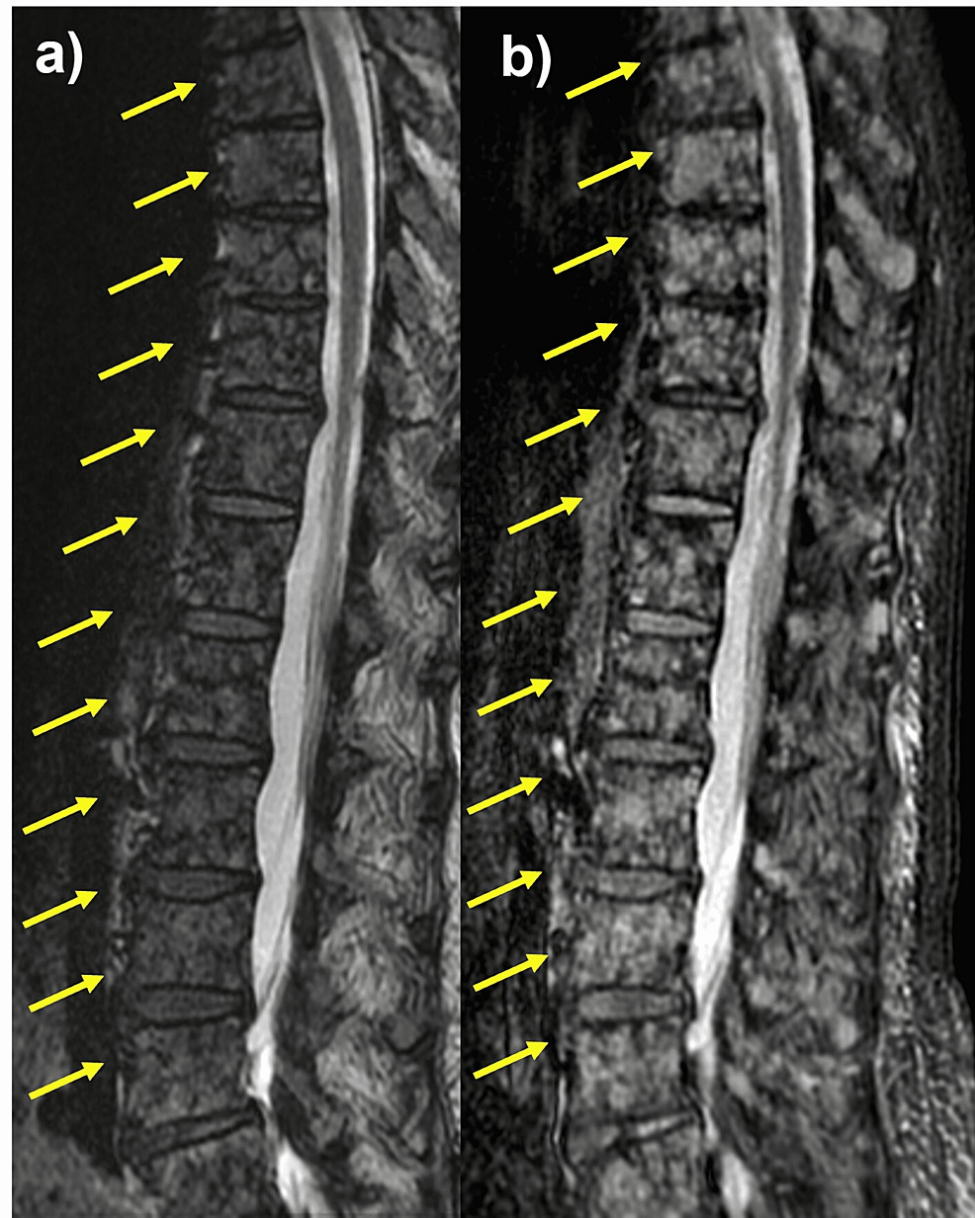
A lumbar spine MRI showing mottled high signal in the lumbar vertebral body a) MRI T2 emphasized image, b) MRI STIR image STIR: Short inversion time inversion-recovery
